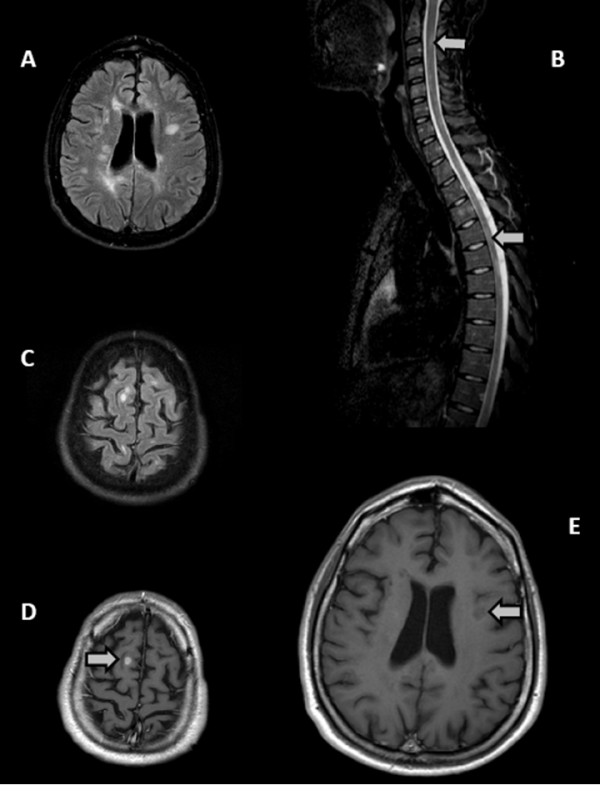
Cranial and spinal MRI before and after treatment with Natalizumab. A. Axial FLAIR (fluid attenuation inversion recovery) scan performed on October 2008 before Natalizumab treatment showing multiple hyperintense MS lesions in the periventricular areas of both hemisheres. B. Sagittal STIR (Short TI Inversion Recovery) sequence of spinal cord performed on October 2008, showing a high number of hyperintense demyelinating lesions in the cervical and dorsal spinal tracts (two representative are indicated by arrows). C. Axial FLAIR scan performed on October 2008 showing hyperintense subcortical MS lesions of both hemisheres. D. After intravenous injection of Gadolinium, an intense contrast enhancement is shown in one subcortical lesion of the right parietal area (arrow). E. Representative axial T1-wheighted scan (periventricular section) after intravenous injection of Gadolinium, performed on October 2009 during natalizumab treatment. Multiple hypointense MS lesions are evident in the periventricular areas of both hemispheres (the largest is indicated by the arrow) with no Gadolinium-enhancement. The MRI evaluation also included T2 and FLAIR scans of the whole brain. Besides the absence of contrast-enhancement, no new or enlarging T2-FLAIR lesions were evident after natalizumab treatment (not shown).
